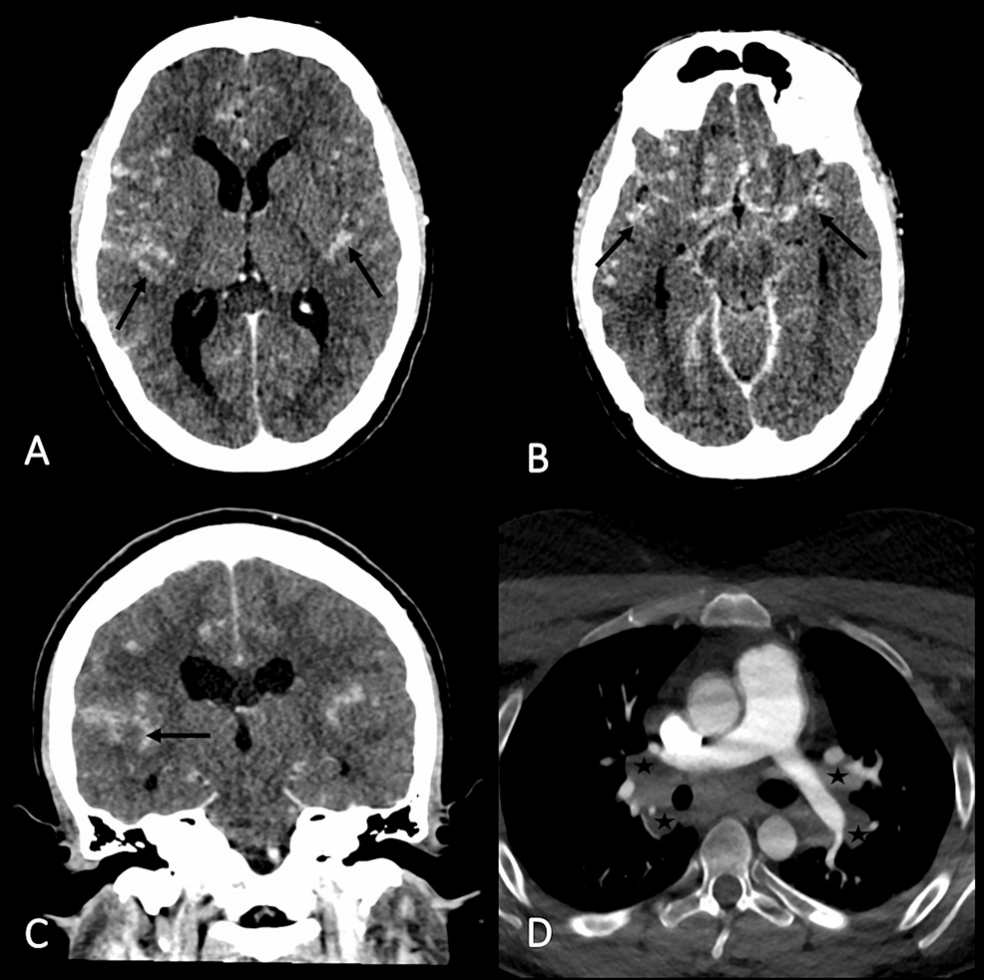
Brain CT scan with nodular leptomeningeal enhancement and lymphadenopathies in the upper part of the mediastinum Initial venous CT showing multiple hyperdensities in cerebral hemispheres predominantly in the temporal lobes corresponding to nodular leptomeningeal enhancement (black arrows) displayed with axial (A,B) and coronal (C) planes. It refers to the presence of nodules or areas of increased contrast enhancement in the leptomeninges. The arterial phase included the upper part of the mediastinum that demonstrated hilar lymphadenopathies (black stars) (D). CT: computed tomography
